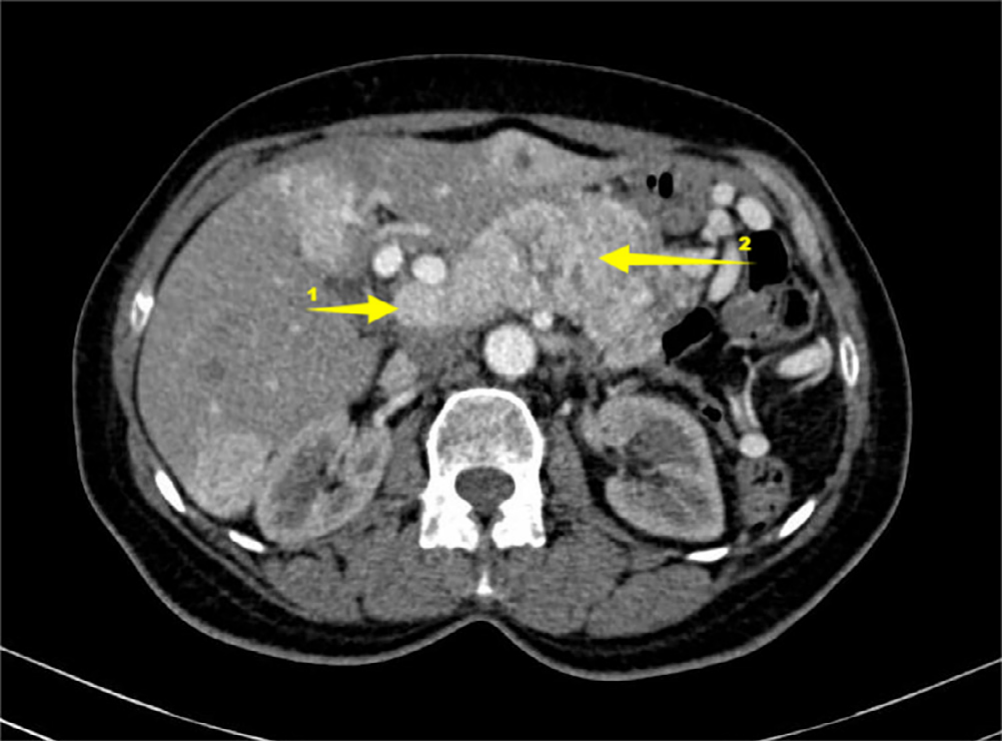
Abdominal injected CT on axial plane. 1 liver tumor. 2 tumoral thrombosis inside portal vein.
